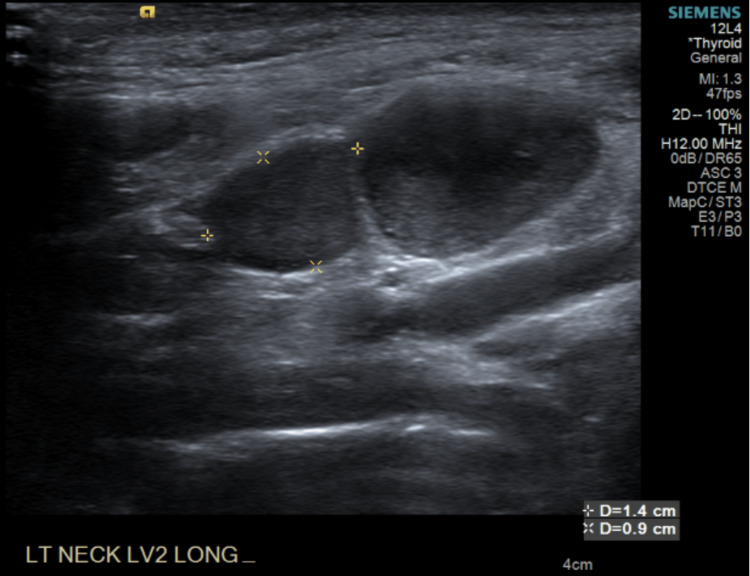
Initial neck ultrasound This ultrasound demonstrated hypoechoic circumscribed nodular structures in the left neck at level 2, the largest of which measured up to 1.9 x 8 2.1 x 1.3 cm. There was no normal fatty hilum seen.
